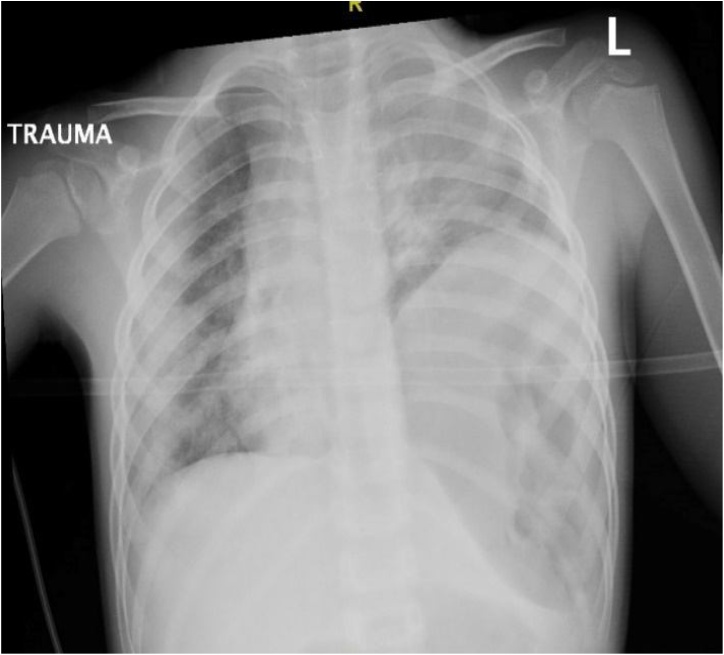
Pre-Operative Chest radiograph.
